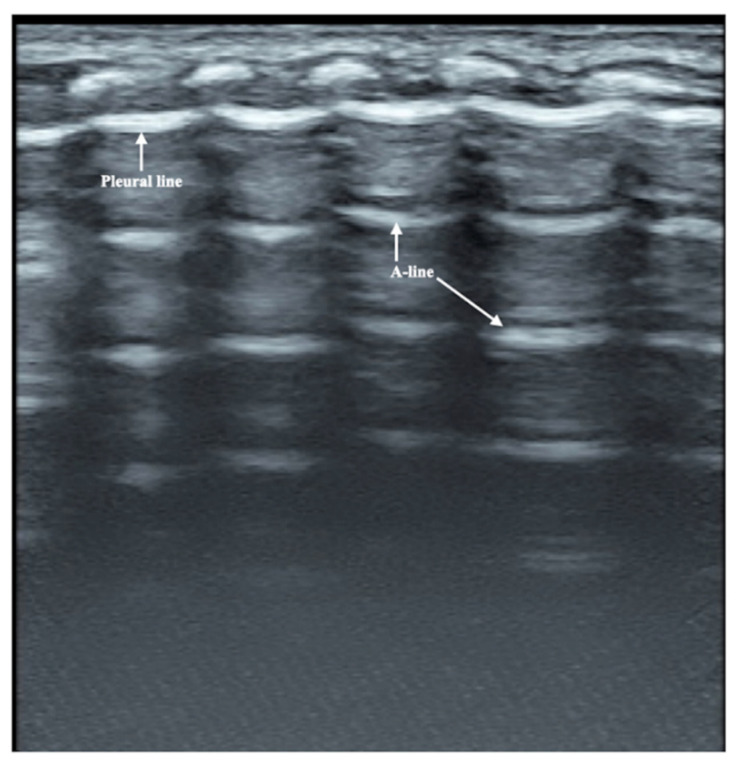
Normal lung ultrasound manifestations. On B-mode ultrasound, the pleural line and A-line show smooth, regular and hyperechoic lines arranged in parallel and equidistant from each other. Together, they form a kind of bamboo-like performance, which is known as the bamboo sign.
